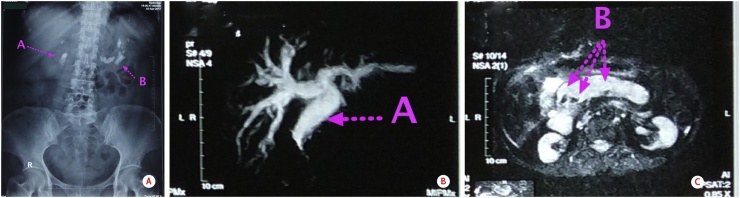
a. Abdominal X-ray; b and c. MRCP showed common bile duct and pancreatic duct dilatation and stone (Arrow A: Stone in the common bile duct, Arrow B: Stones in the pancreatic duct).
